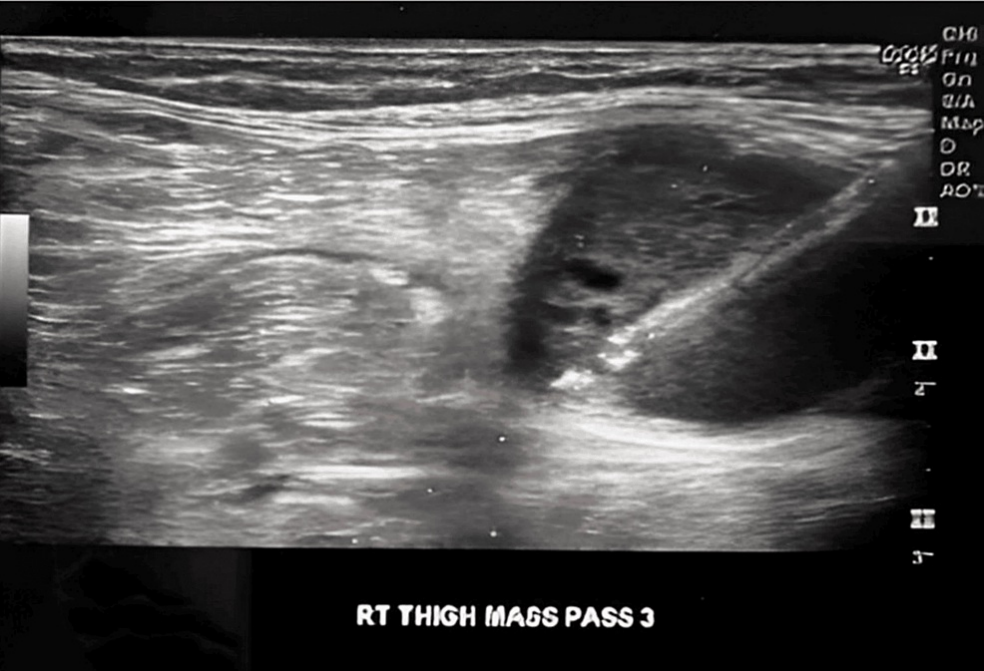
Ultrasound image while obtaining a biopsy of the sartorius muscle
